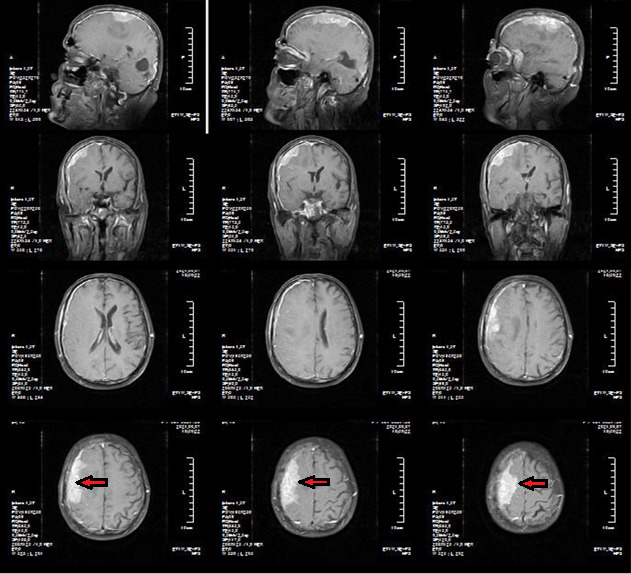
Brain MRI obtained in sagittal, axial, and coronal planes. On post‐contrast MRI images, there are diffuse enhancement of leptomeninges. There is also an enhancing mass at the upper aspect of the parietal lobe (red arrow)
